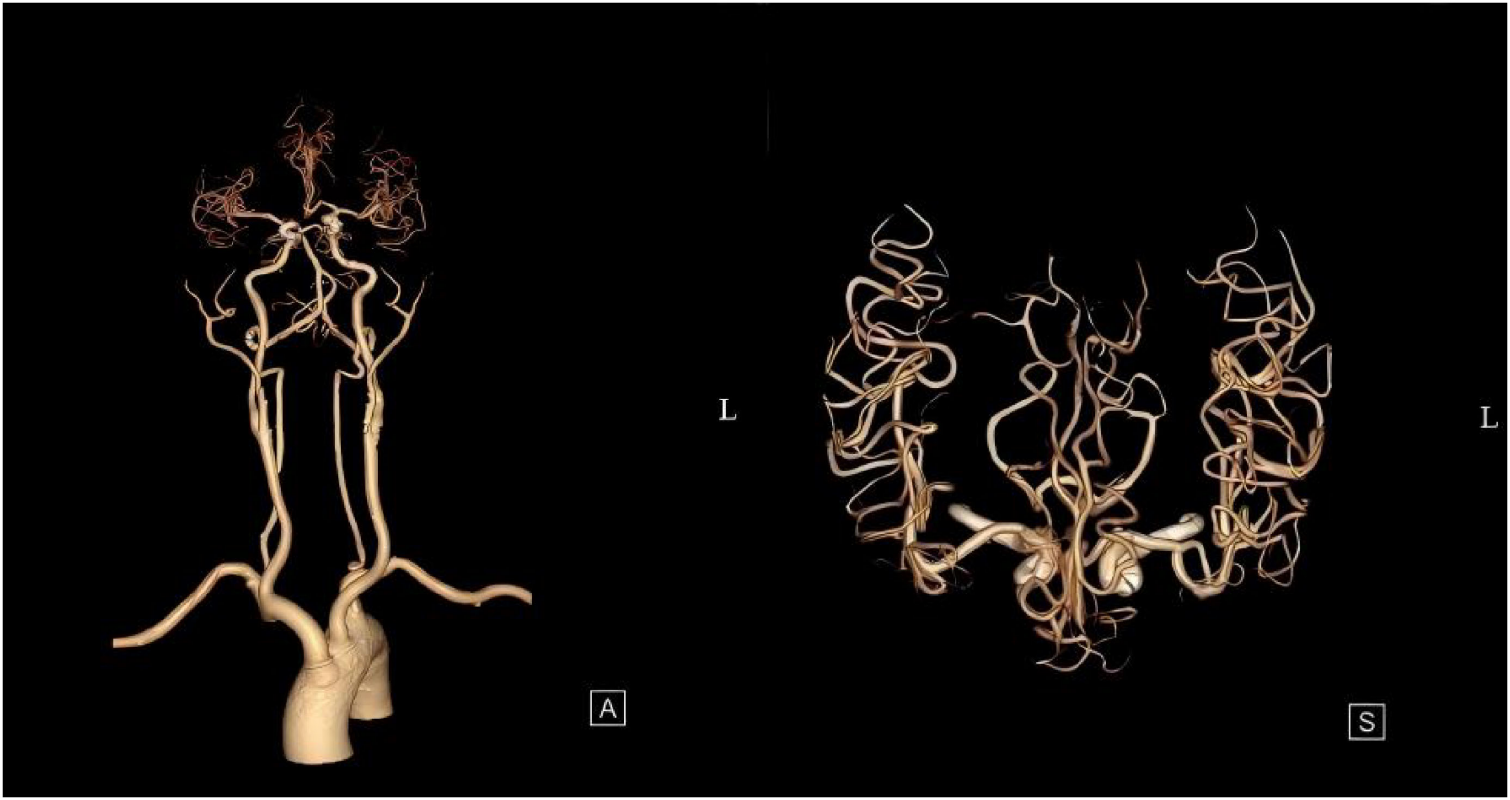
Cranial vascular CTA suggestive of cerebral atherosclerosis.
